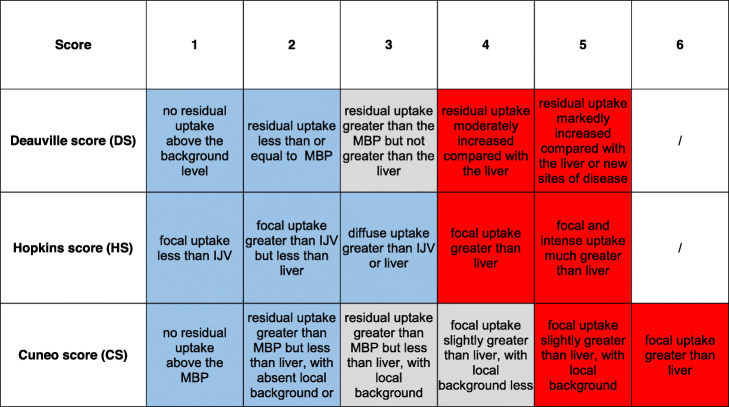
Standardized reporting criteria used to assess FDG-PET/CT scans (Deauville, Hopkins, and Cuneo scores)
